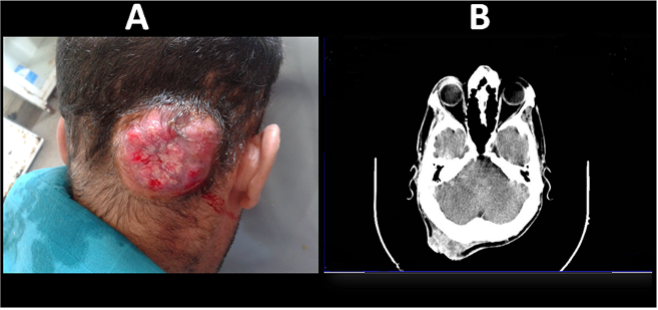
Skin carcinoma: Macroscopic view (A), and cranial computed tomography scan (B). Exophytic lesion of the scalp.
